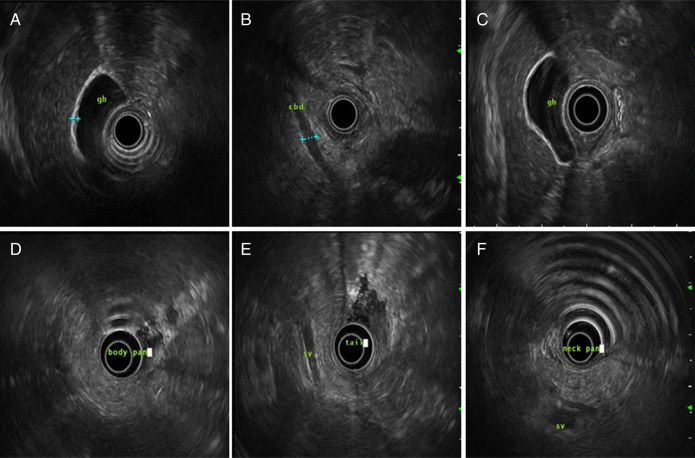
(A–C) EGD and EUS revealed a nondilated common bile duct, normal gallbladder, without stones/sludge. (D–F) EGD and EUS show normal pancreas. EGD, esophagogastroduodenoscopy; EUS, endoscopic ultrasound.
